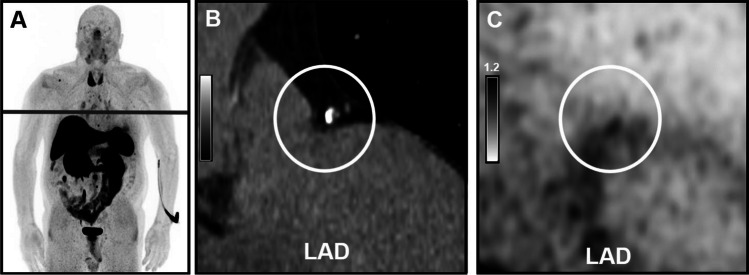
Example image revealing [^68^ Ga]Ga-DOTA-TOC uptake in calcified LAD plaque. A 78 y/o male patient with neuroendocrine tumor (NET) of the ileum was referred for [^68^ Ga]Ga-DOTA-TOC LAFOV PET/CT. An unknown calcified plaque with significant [^68^ Ga]Ga-TOTA-TOC uptake (SUV_max_: 1.75, TBR: 2.50) was detected in the LAD. A shows the maximum intensity projection (MIP), B shows a coronal CT image, and C shows the coronal PET image
